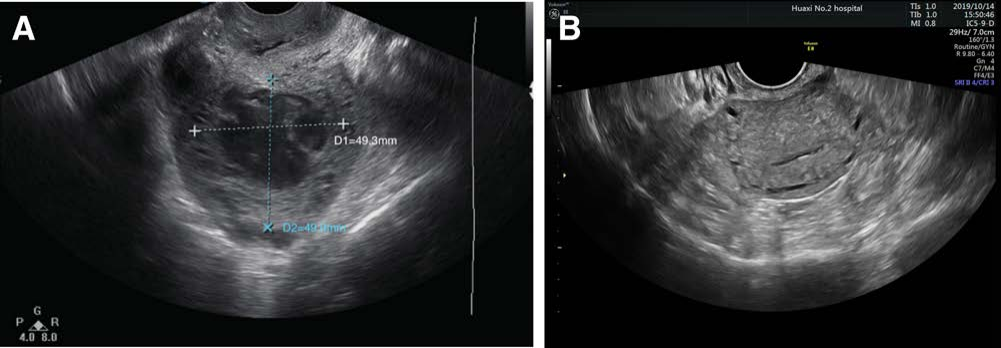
Comparison of transvaginal ultrasound. (A) A significant enlargement of corpus uteri with an occupation of 49 mm × 49 mm × 46 mm in size. Myometrium of the anterior wall was invaded with unclear boundary before chemotherapy. (B) Transvaginal ultrasound showed uterus shrunk and occupation within myometrium disappeared after chemotherapy.
